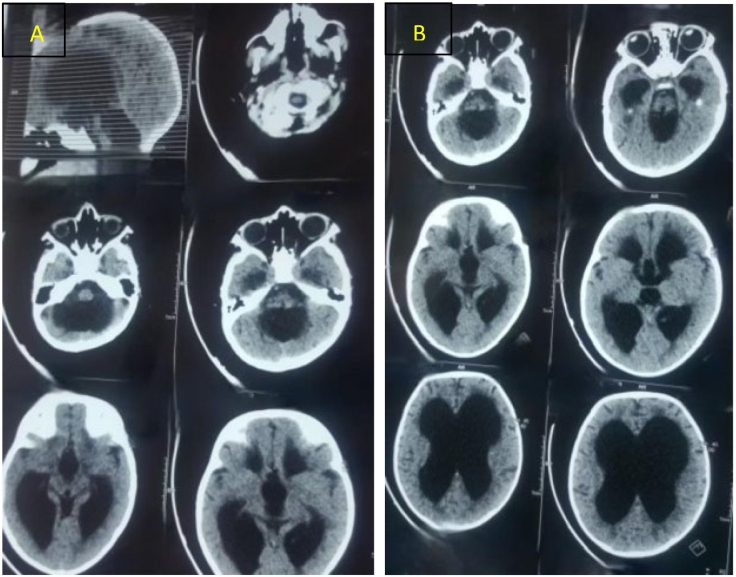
(A, B): Axial and sagittal brain CT scans demonstrating the absence of the cerebellar vermis and associated hydrocephalus.
